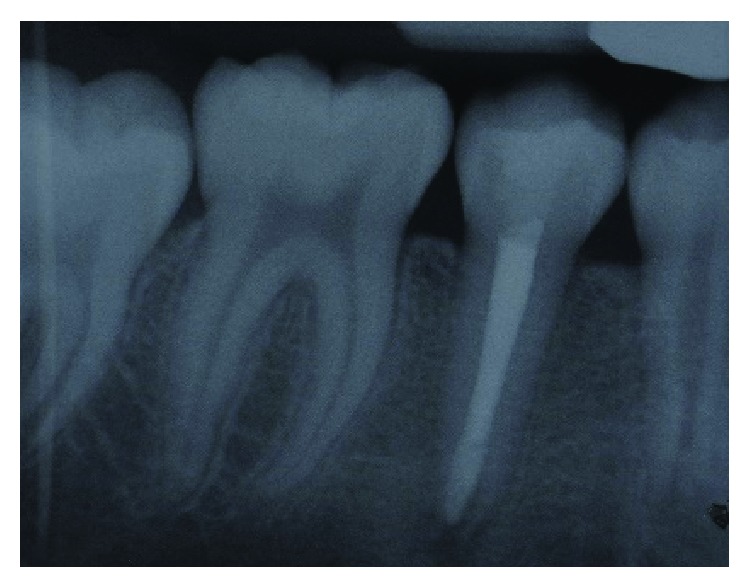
Periapical radiograph of tooth 45 after root canal obturation with thermoplasticised gutta-percha.
